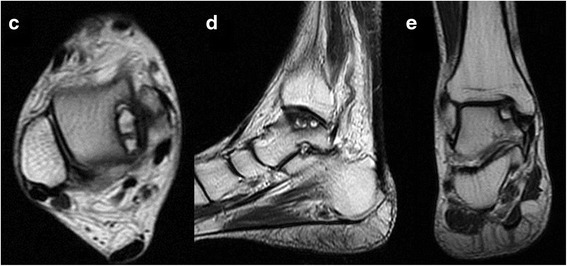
A 63 year old female with concomitant large OLTs and varus ankle malalignment. c-e A large subchondral cyst was found with high signals at the medial talar shoulder on T2-weighted images
